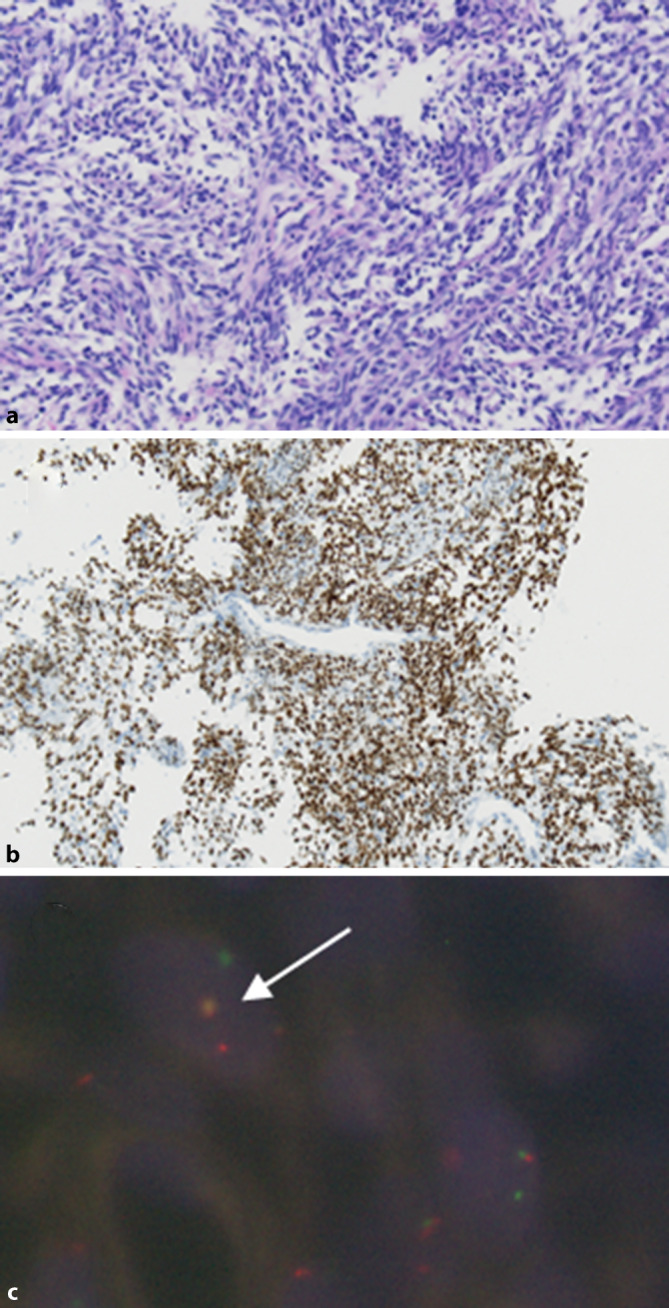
Es erfolgten drei CT-Aufnahmen. In der initialen Bildgebung (vor Induktion der Chemotherapie) stellte sich ein ulzerierender Tumor der Magenkorpushinterwand großkurvaturseitig dar (Abb. 2a). Dieser war im Verlauf (Abb. 2b) und nach beendeter Chemotherapie (Abb. 2c) größenregredient.
